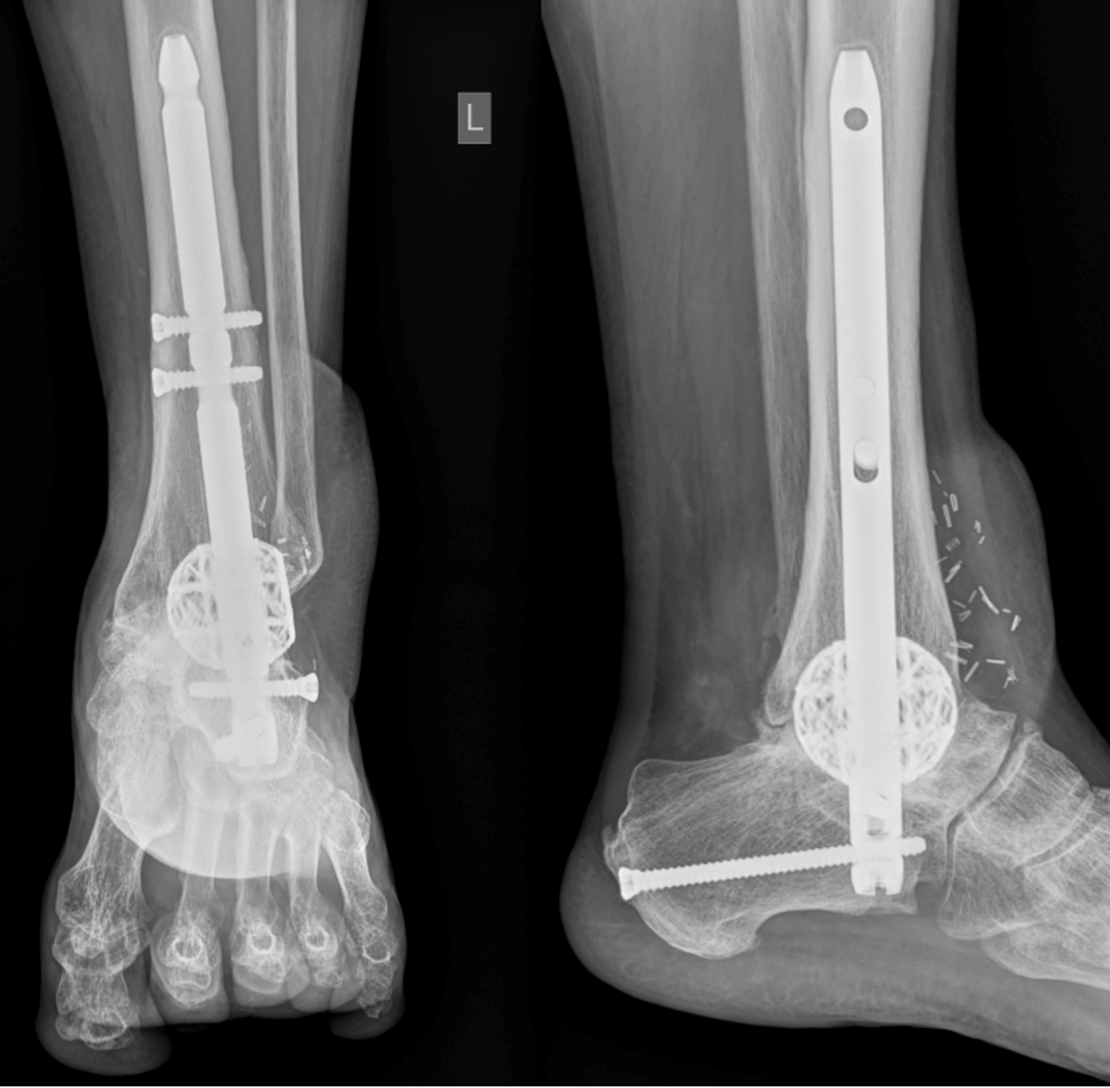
Anterior-posterior (left) and lateral (right) X-rays of a successful implant that did not require revision surgery. The patient is a 56-year-old polytrauma patient who underwent a custom 3D-printed cage with a tibiotalocalcaneal nail following washout and cement spacer insert.
